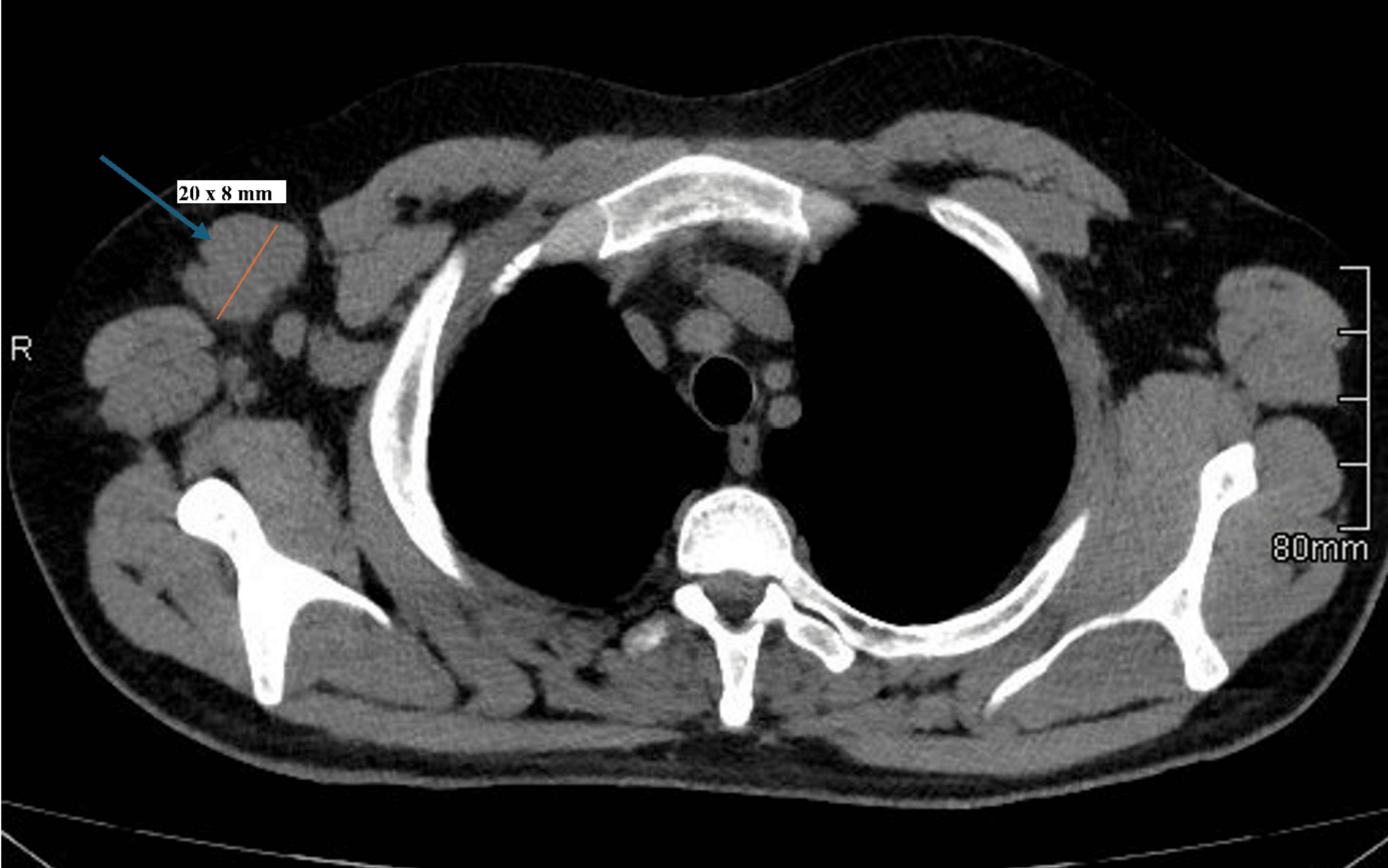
Axial CT scan of the chest showing the largest right axillary lymph node, measuring 20 x 8 mm (blue arrow).
